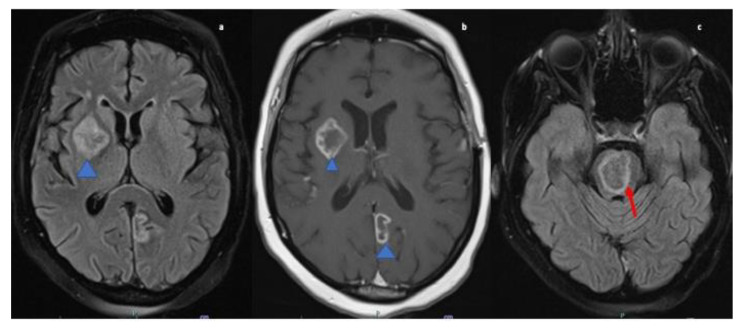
MRI Brain axial fluid-attenuated inversion recovery (FLAIR; (a)) & T1-weighted post contrast image (b) reveals hyperintense lesion in right gangliocapuslar region (blue arrowhead; (a)) with corresponding heterogenous peripheral ring enhancement, in addition to enhancement in the left paramedian occipital lobe (blue arrow head; (b)). FLAIR image (c) reveals circumscribed intra-axial parenchymal lesion in the pons (red arrow).
